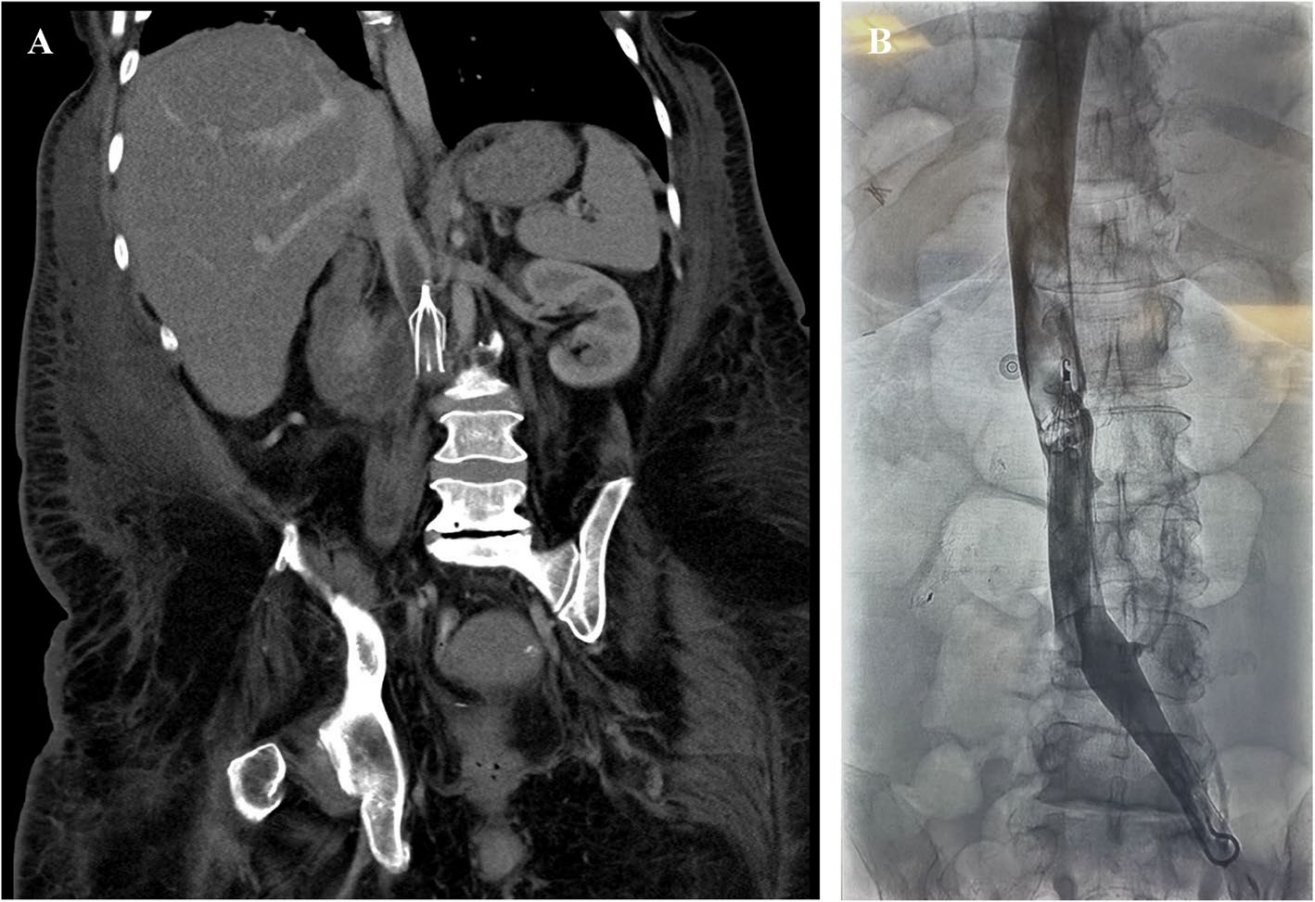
A CT abdomen with contrast and B fluoroscopy contrast study showing an adherent nonocclusive thrombus within and above an indwelling IVC filter
